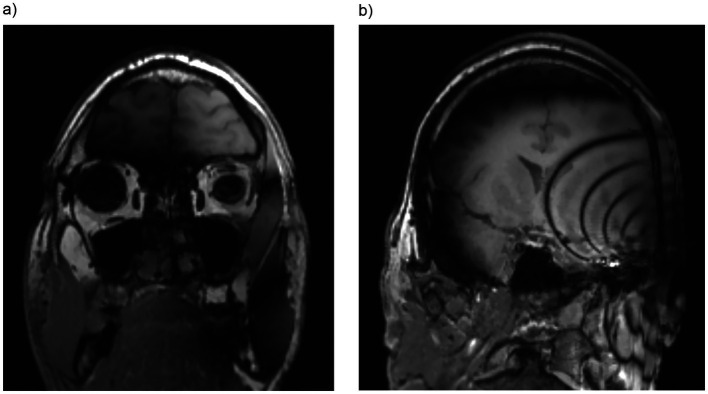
Both maxillary sinuses are fully visible and assessable in T1-weighted sequence (a) in a unilateral CI with a magnet positioned at a 90° angle. Furthermore, T1-weighted sequence (b) in a unilateral CI with a magnet positioned at a 120° angle demonstrating that the sphenoid sinus is only partially assessable. Coronal images are reconstructed from the volumetric T1 MP-RAGE sequence.
